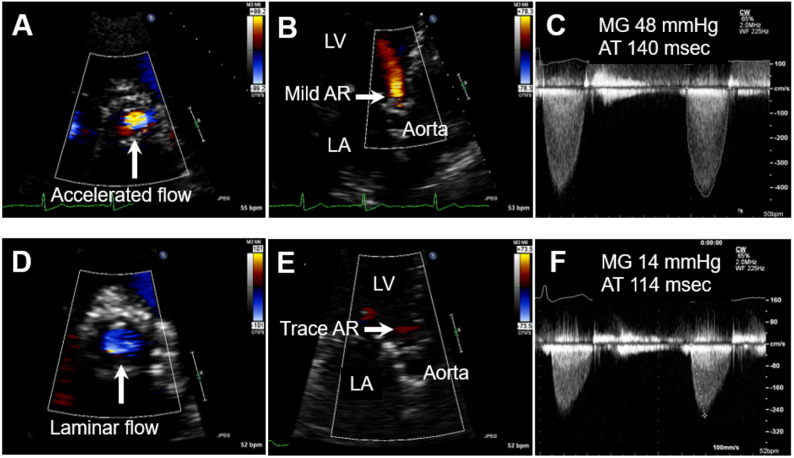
Transthoracic echocardiogram with CW Doppler before (A–C) and after (D–F) warfarin course (note virtually identical CW Doppler settings). (A) Short axis view demonstrating significant flow acceleration through the aortic prosthesis; (B) apical-axis view demonstrating mild aortic regurgitation (LV: left ventricle, LA: left atrium); (C) severely increased mean transvalvular gradient (MG) of 48 mmHg with prolonged acceleration time (AT) 140 msec. (D) Laminar flow through the aortic valve; (E) near disappearance of aortic regurgitation; (F) decrease in MG to 14 mmHg and AT to 114 msec.
